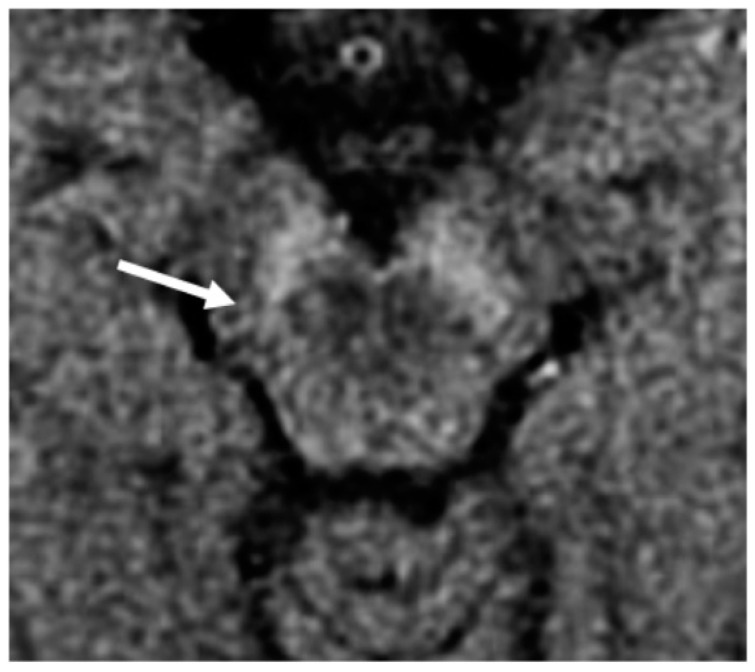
NM-MRI sequence with an explicit MT preparation pulse, scanned with a 1.5T MR scanner at the level of the SN in a PD patient with asymmetrical motor symptoms onset: the loss of hyperintensity in the posterolateral aspect of the right SN (arrow) correlated well with the clinical presentation.
